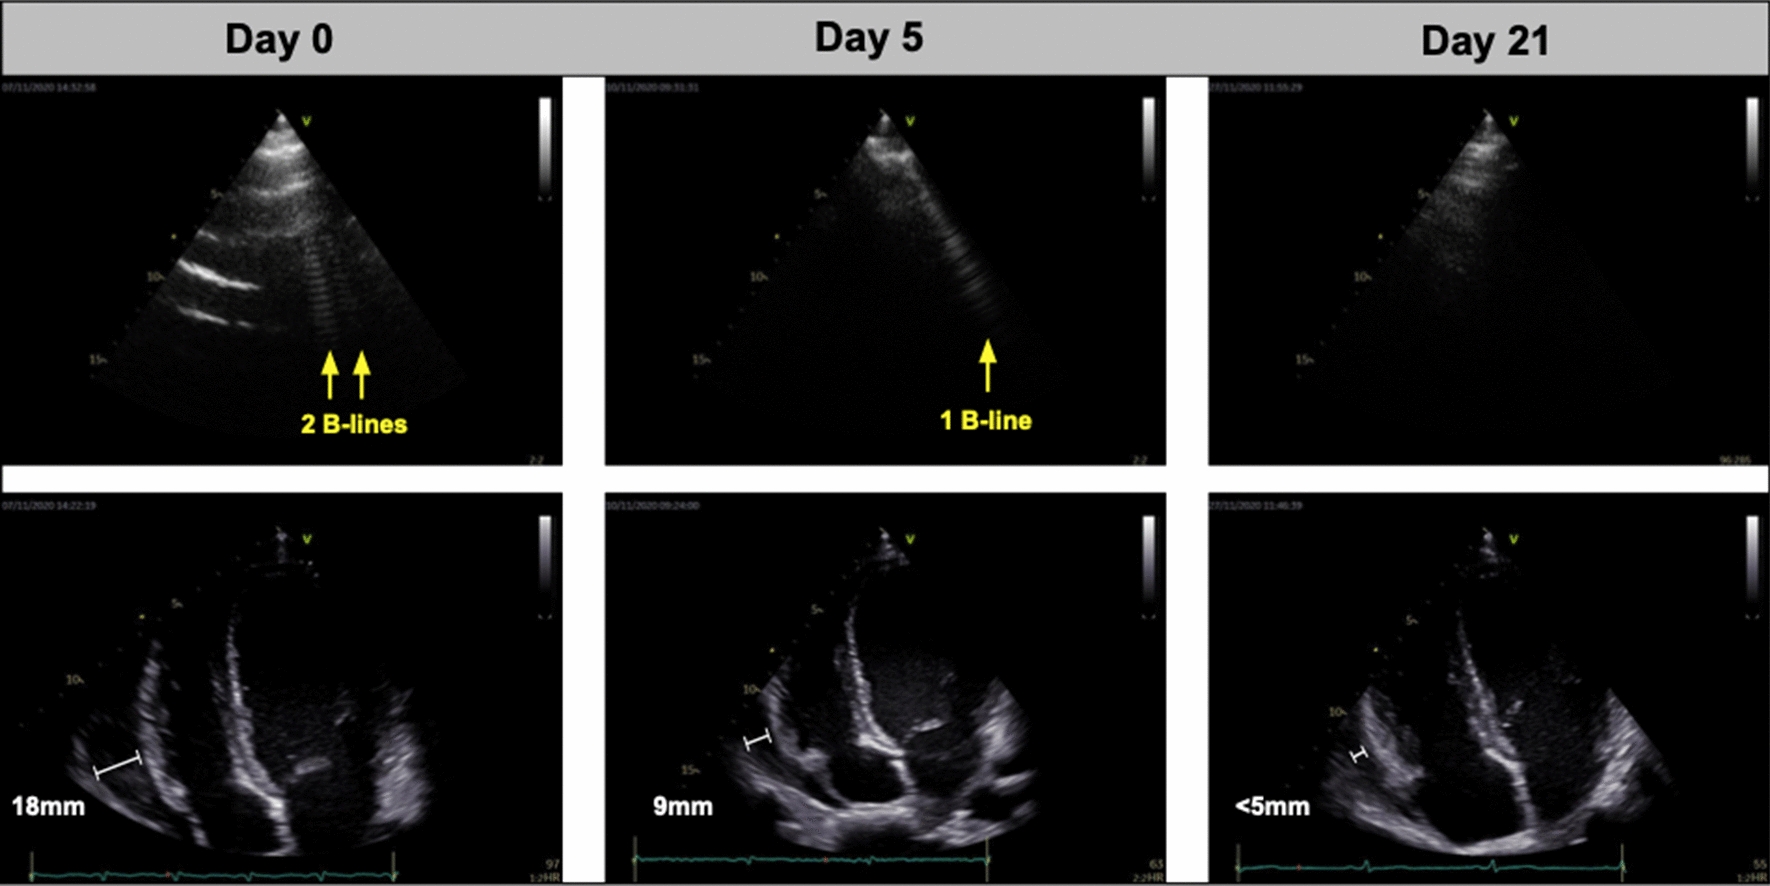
Images of cardiopulmonary complications in malaria by day 0, 5 and 21. Upper row shows B-lines by lung ultrasound (white artefacts extending from the pleura) and the lower row displays pericardial effusion measured next to the right ventricle
